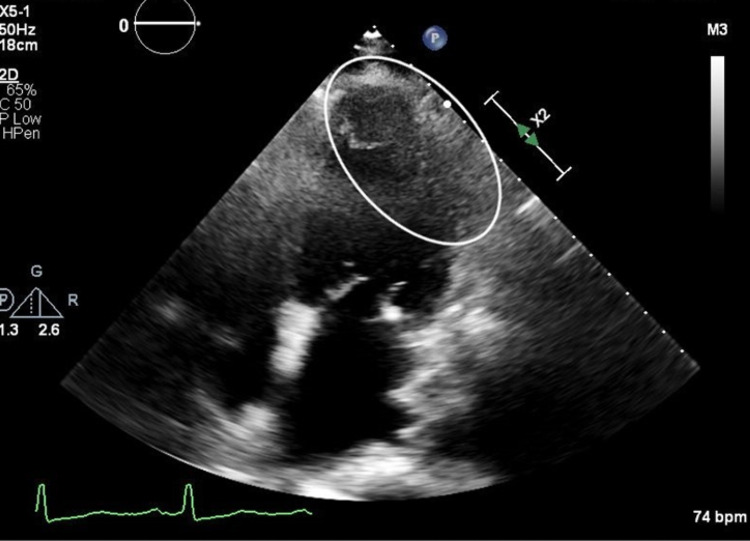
Transthoracic echocardiogram showing a 2.2 × 2.3 cm globular structure in left ventricular apex with an abnormal appearance of the apical lateral segment.
